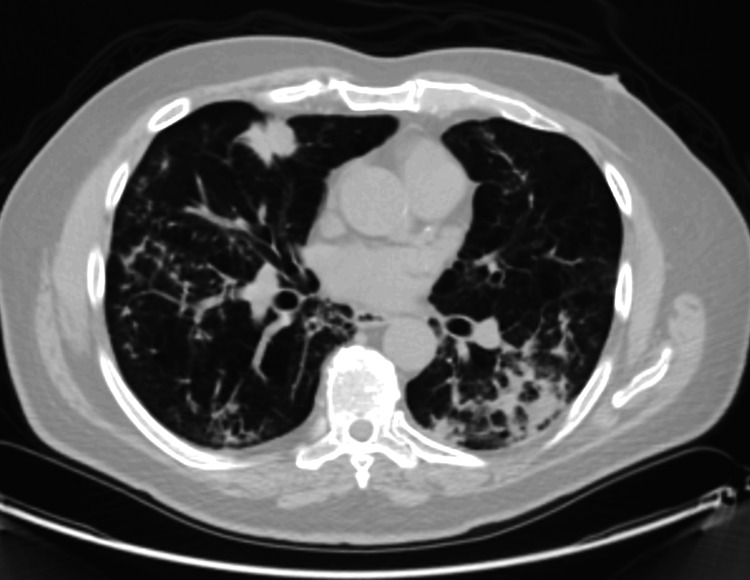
Chest CT November 2023: Before Treatment Abbreviations: CT, computed tomography
